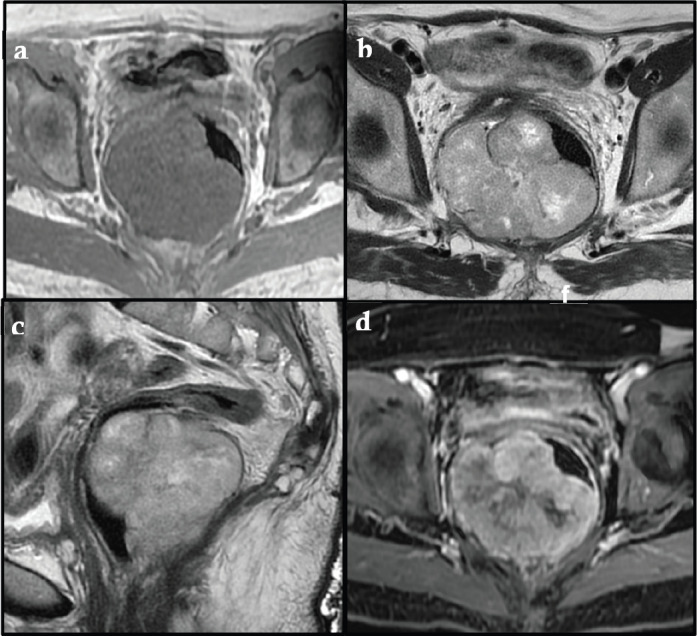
(a, b, c): Axial T1-weighted MR and Axial, Sagittal and Coronal T2-weighted MR respectively showed a large isointense mass in T1, iso-hyperintense in T2, with well-defined edges located in the pelvic region. The posterior and right lateral walls of the median and lower rectum were involved, attached to the mesorectum and levator ani muscle with a predominantly exophytic component and an intraluminal component. MR showed a tumour mass compressing and displacing the rectum to the left and the uterus superiorly. No lymphadenopathy was observed. (d). Post-contrast Axial Fat-Suppressed T1-weighted MR shows a solid component of the mass-enhanced heterogeneously.
